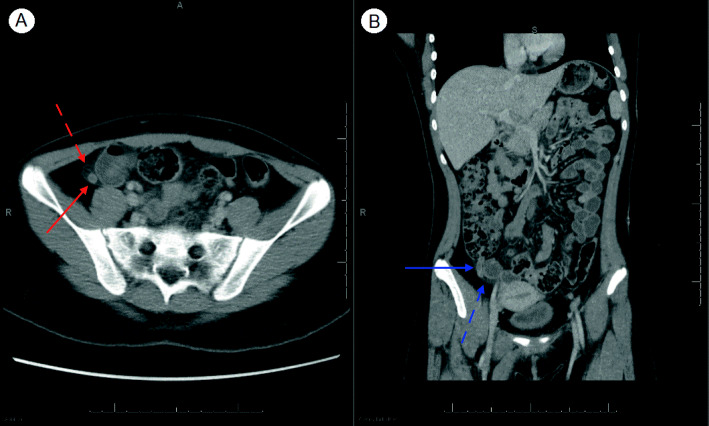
CT abdomen/pelvis. (A) Appendix (red solid arrow) wall thickness > 3mm. Lack of luminal air, appendix fluid depth > 2.6mm, and hyperdensity. Red dashed arrow shows peri-appendiceal fat stranding. (B) A diameter of 8mm (blue solid arrow) with inflammatory fat stranding (blue dashed arrow), most pronounced around the cecum
